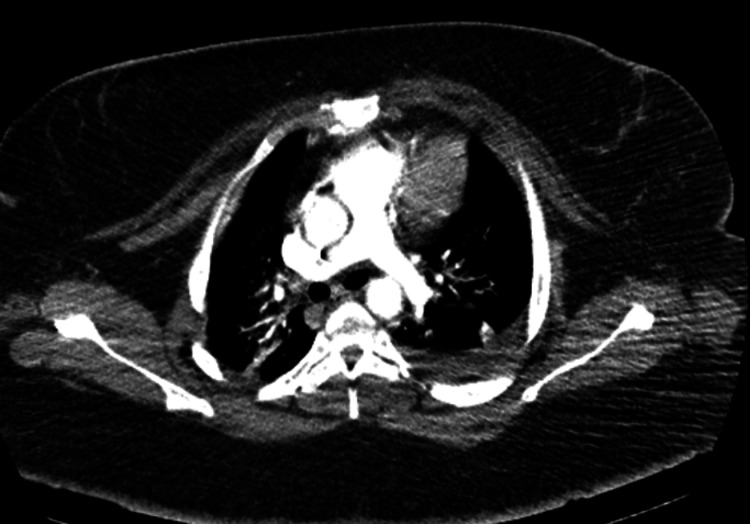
Normal CT pulmonary angiography.
